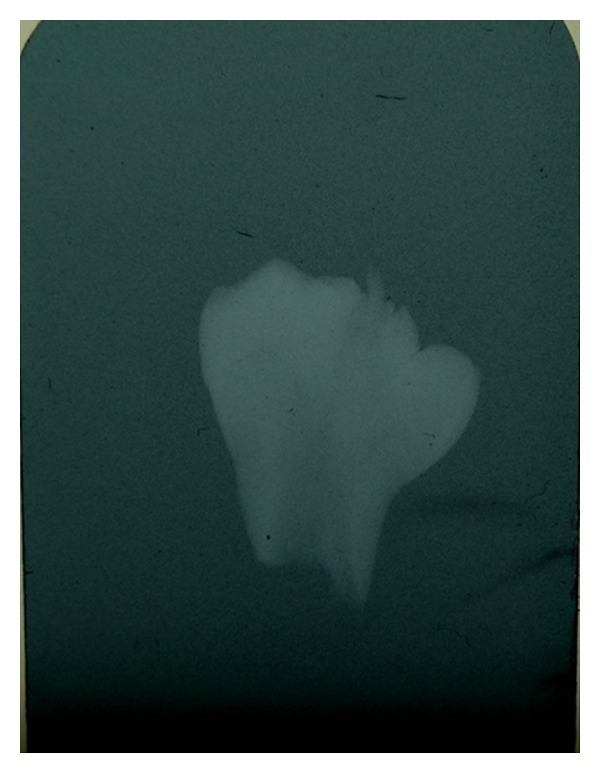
Radiograph showing two separate pulp chambers and root canals of extracted fused supernumerary teeth.
